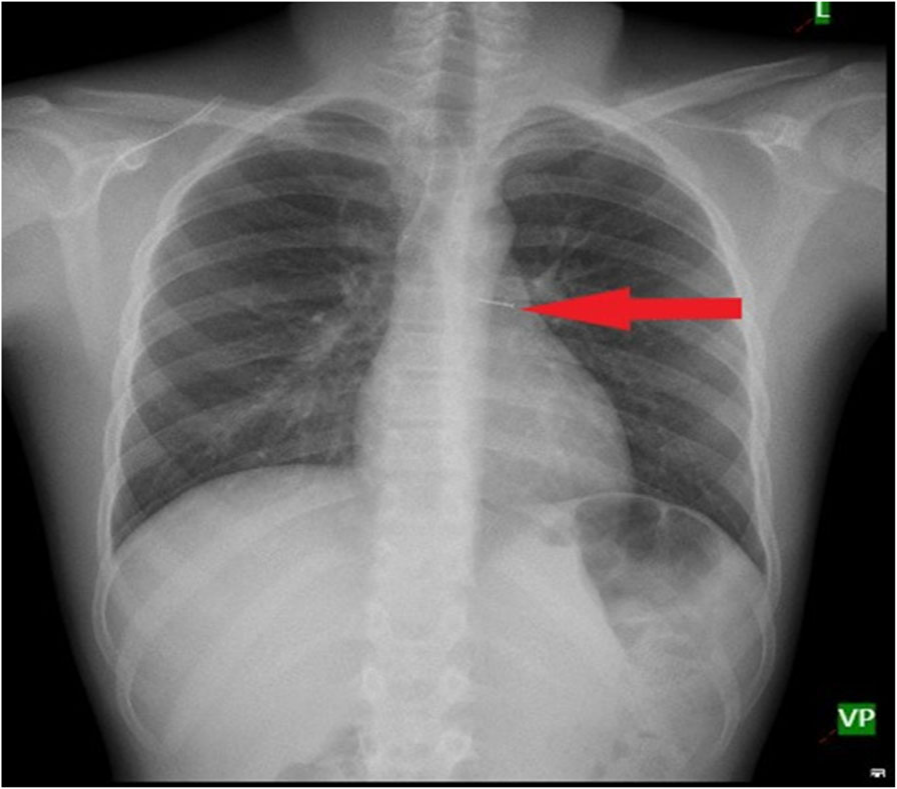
Chest x-ray showing pin in the left main bronchus
